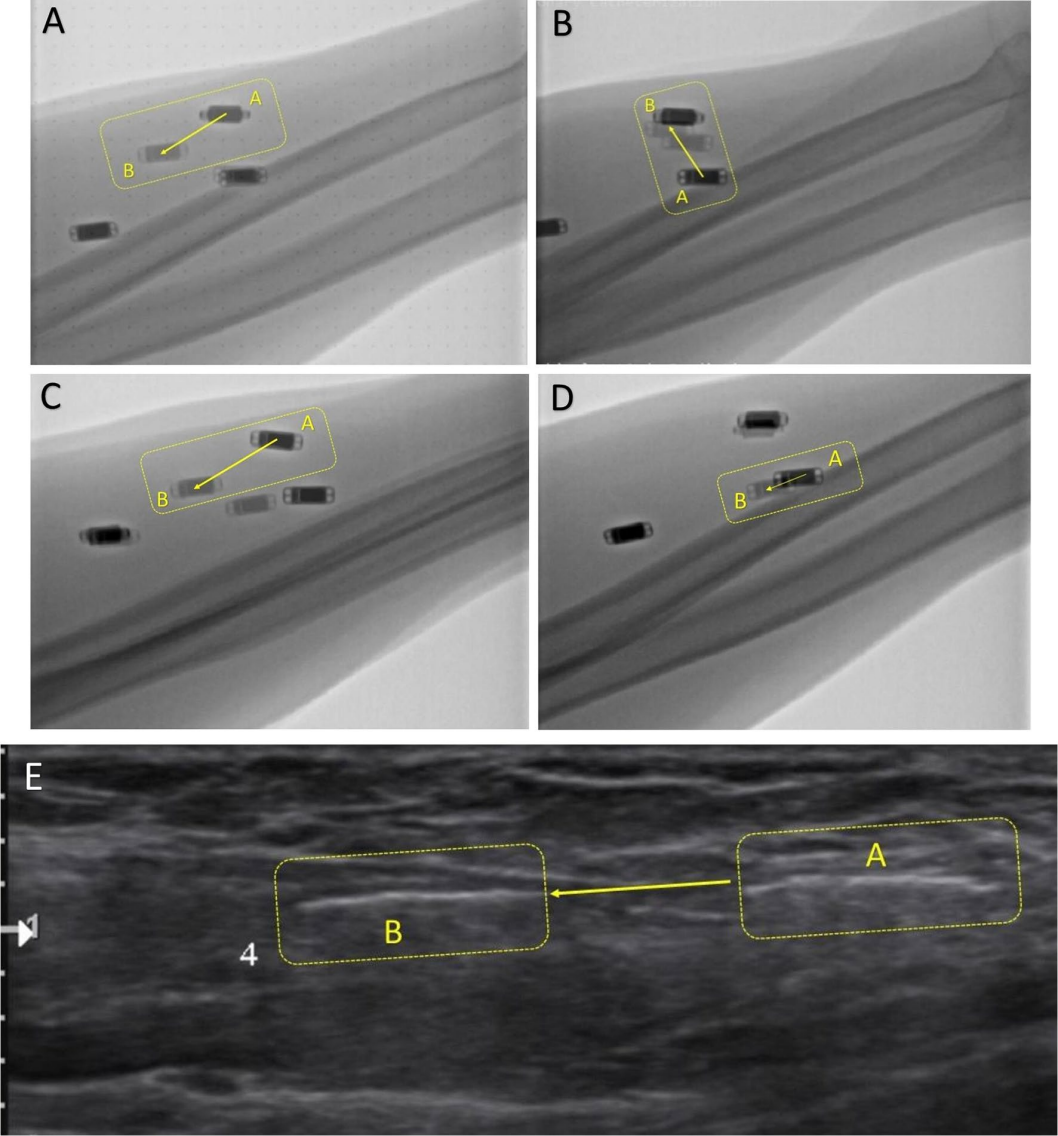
Some of the independent tags’ movements in fluoroscopy and ultrasound: Tag 1 displacement with 4th and 5th fingers Flex-Ext in fluoroscopy (A), Tag 3 displacement with the forearm supination-pronation in fluoroscopy (B), Tag 1 displacement with grasp and release in fluoroscopy (C), Tag 3 displacement with 2nd finger Flex-Ext in fluoroscopy (D); Tag 1 displacement with 4th and 5th fingers Flex-Ext in ultrasound (E).
